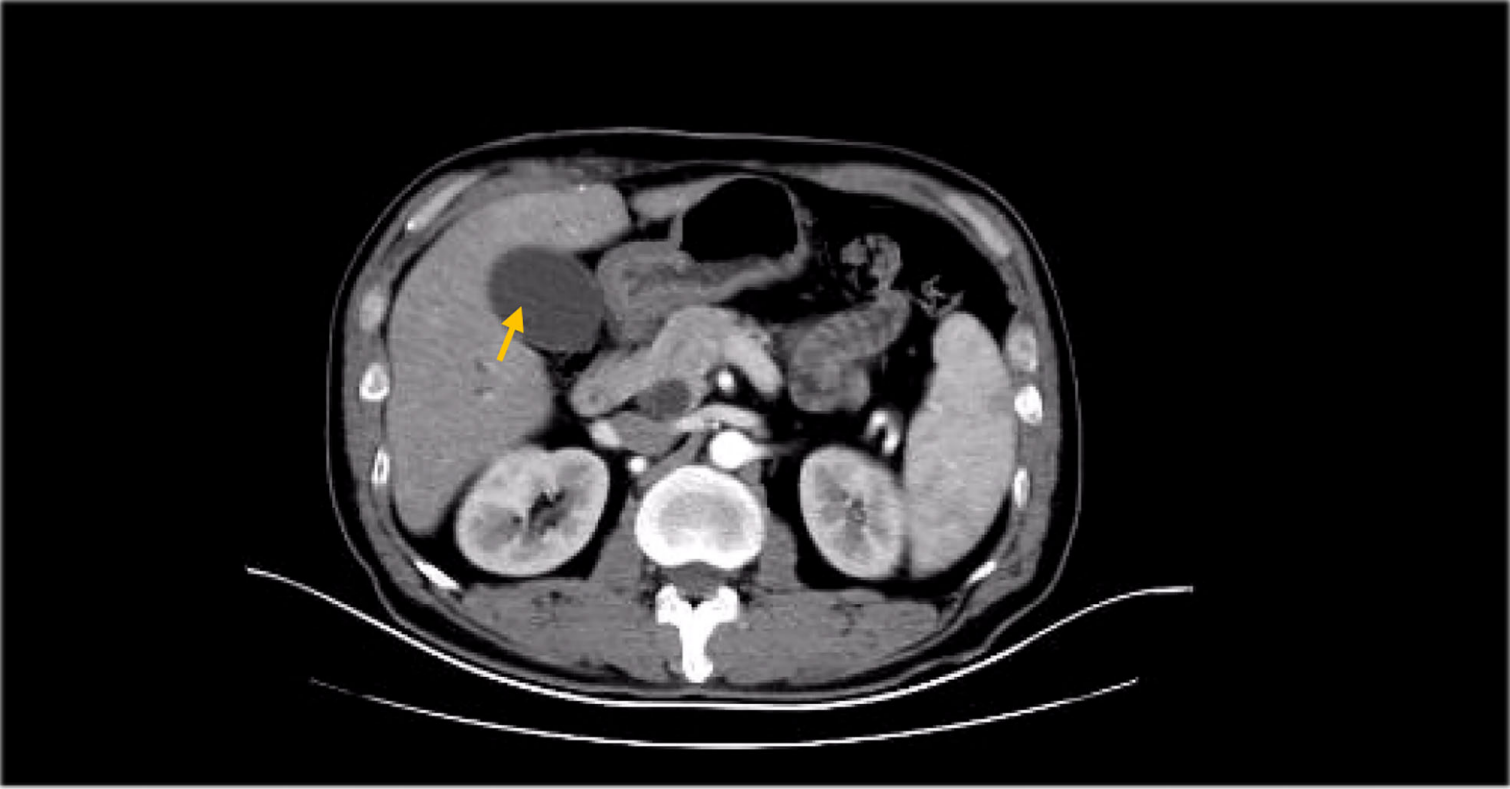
CT (enlargement of gallbladder due to biliary obstruction).
